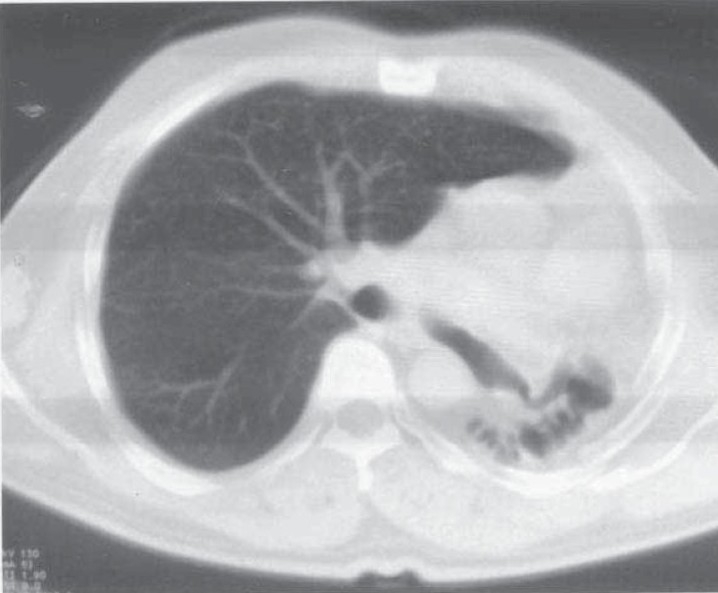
CT chest showing minimal aerated lung on the left side with herniation of the right lung.
